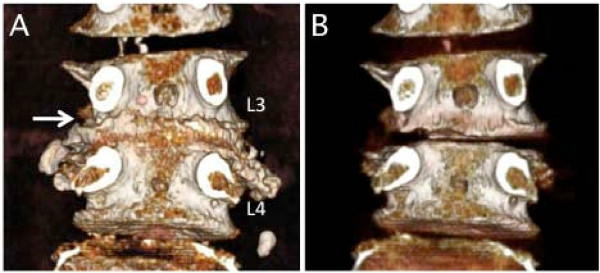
Case 2. A three-dementional CT-discogram obtained from a 69-year-old female who presented severe left leg pain. A protruded disc (A) was recognized by comparing the plane 3-D CT scan (B).
